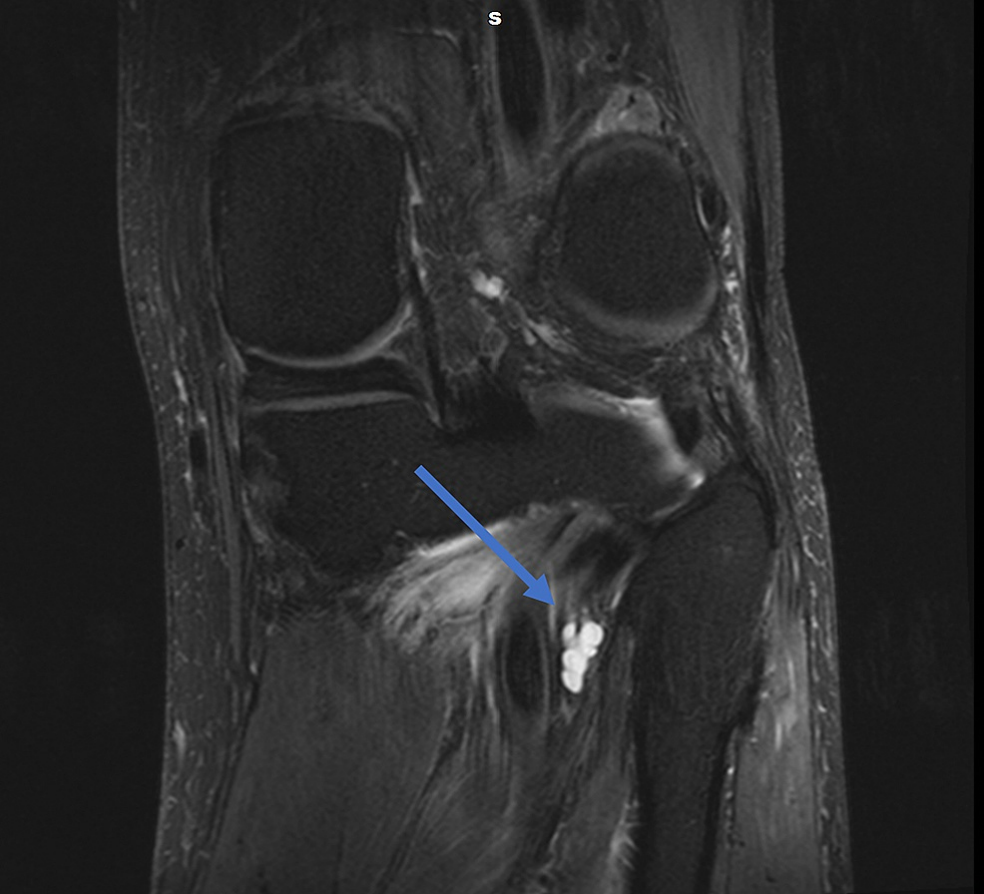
Coronal T2-weighted TSE fat-saturated image demonstrates demonstrating tubular cysts that extend into the popliteus tendon TSE, turbo spin echo
